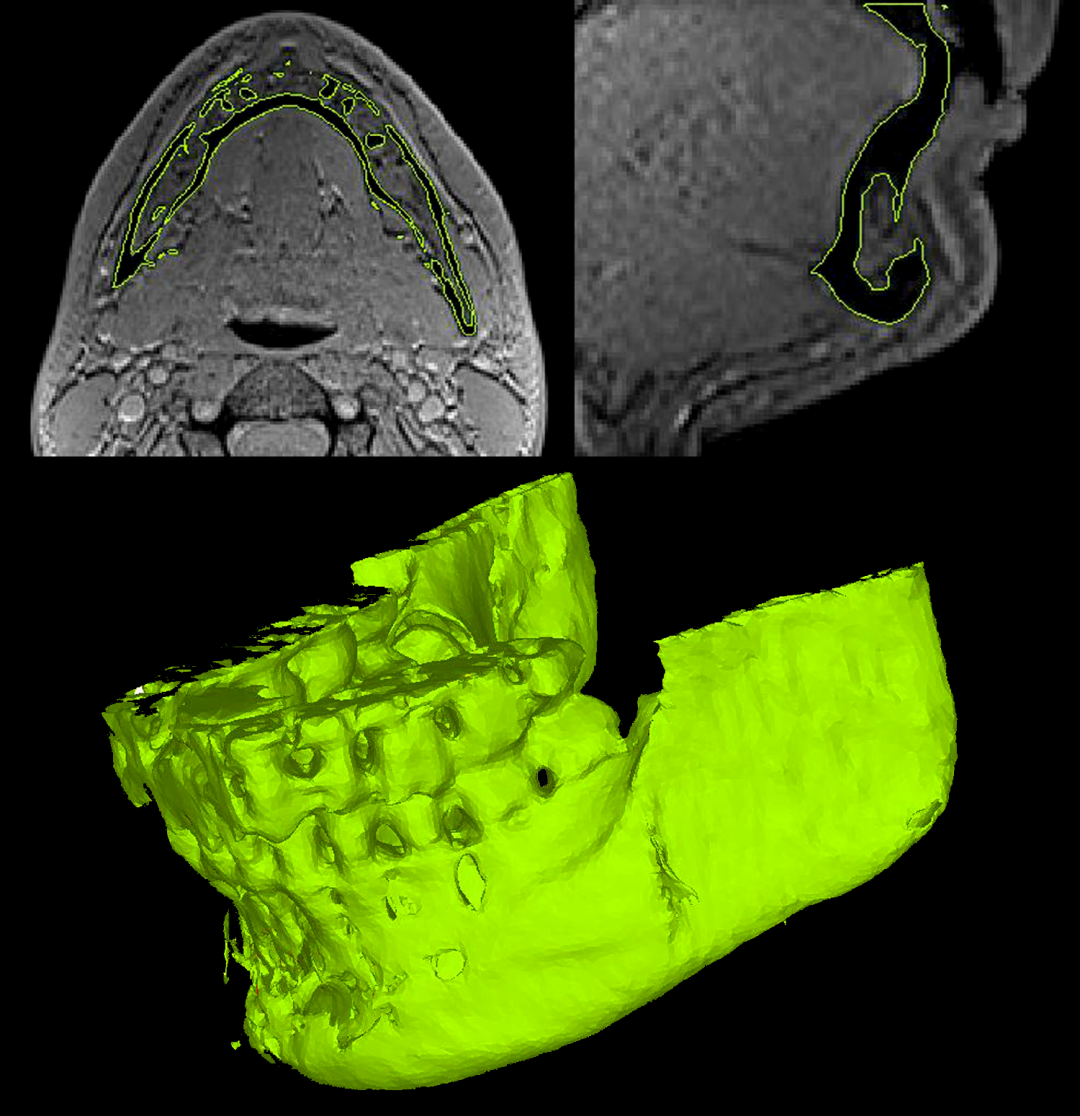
Impaired segmentation quality of the mental region. The axial and sagittal slices and the 3D model derived from the standard Black Bone MRI sequence (with a flip angle of 2°) show the impaired segmentation quality of the mental region. The interruption in the black boundary (cortical bone) creating the virtual holes in the model is visible in the slices.
